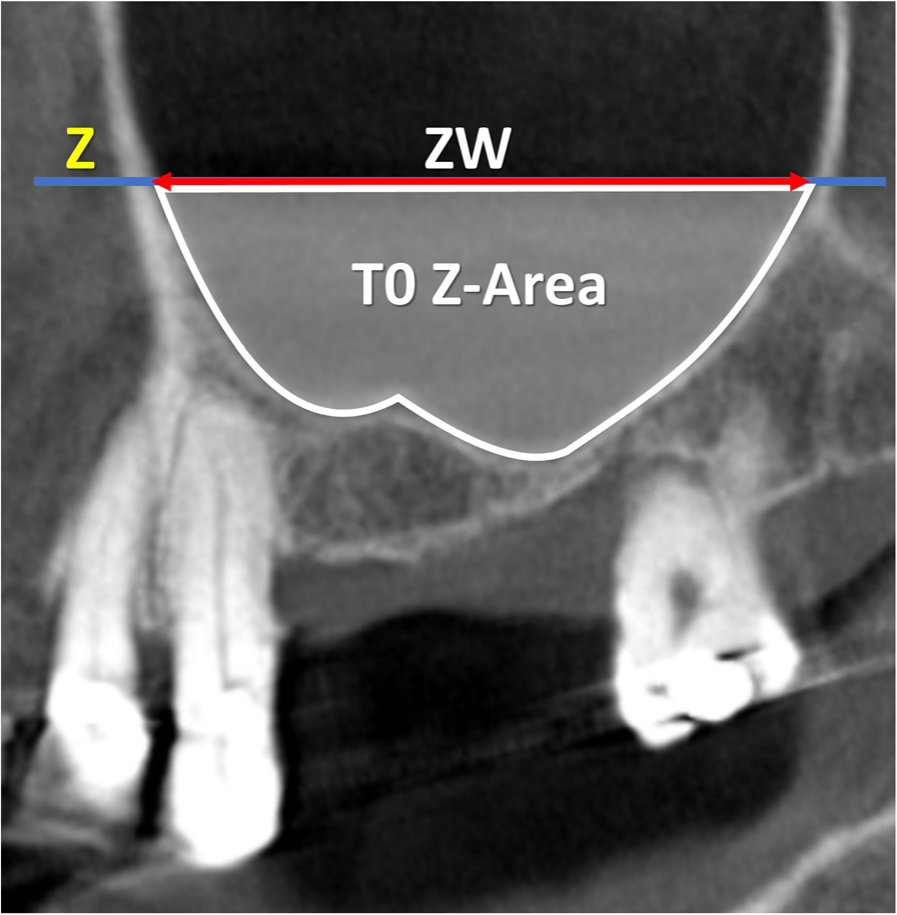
T0, lateral view of a no-membrane CBCT; Z, line drawn following the floor of the nose; ZW (sinus extension), distance evaluated on the line Z between the two intersection points with the mesial and distal sinus bone walls; T0 Z-Area, area delimited by the sinus bone walls and the line Z
